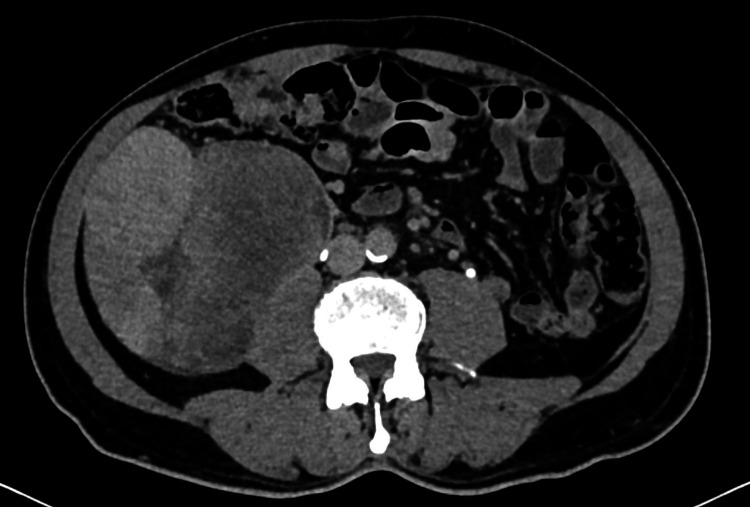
Computed tomography scan, axial section showing a huge mass in the right anterior pararenal space, abutting inferior vena cava
